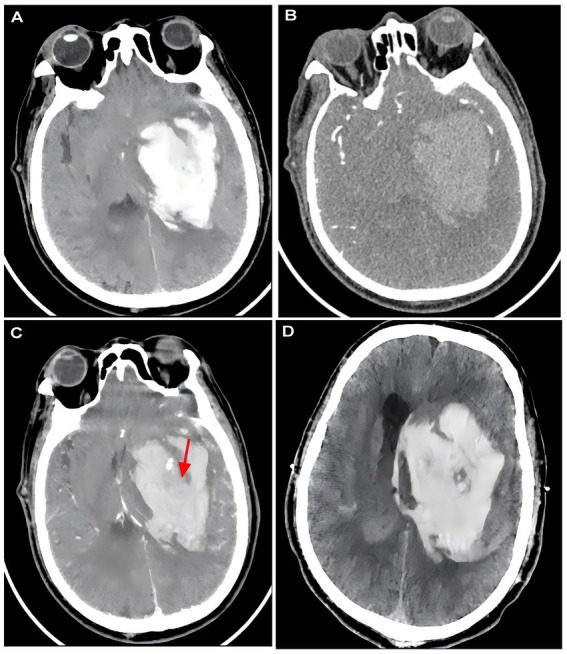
A 77-year-old man with hemorrhage in the left basal ganglia and frontal parietal lobe. The onset time was 1:26 on 20 September, and the time of arrival at the emergency department of our hospital was 8:36. (A,B) First CT and enhanced CT were performed at 8:50. The hematoma volume was 118.50 mL, and the spot sign was negative. (C) Sequential CT scan at 8:52, brush sign was positive, and morphology was continuous (red arrow); and (D) re-examined CT at 11:47, the hematoma volume was 160.00 mL, which showed obvious hematoma expansion.
